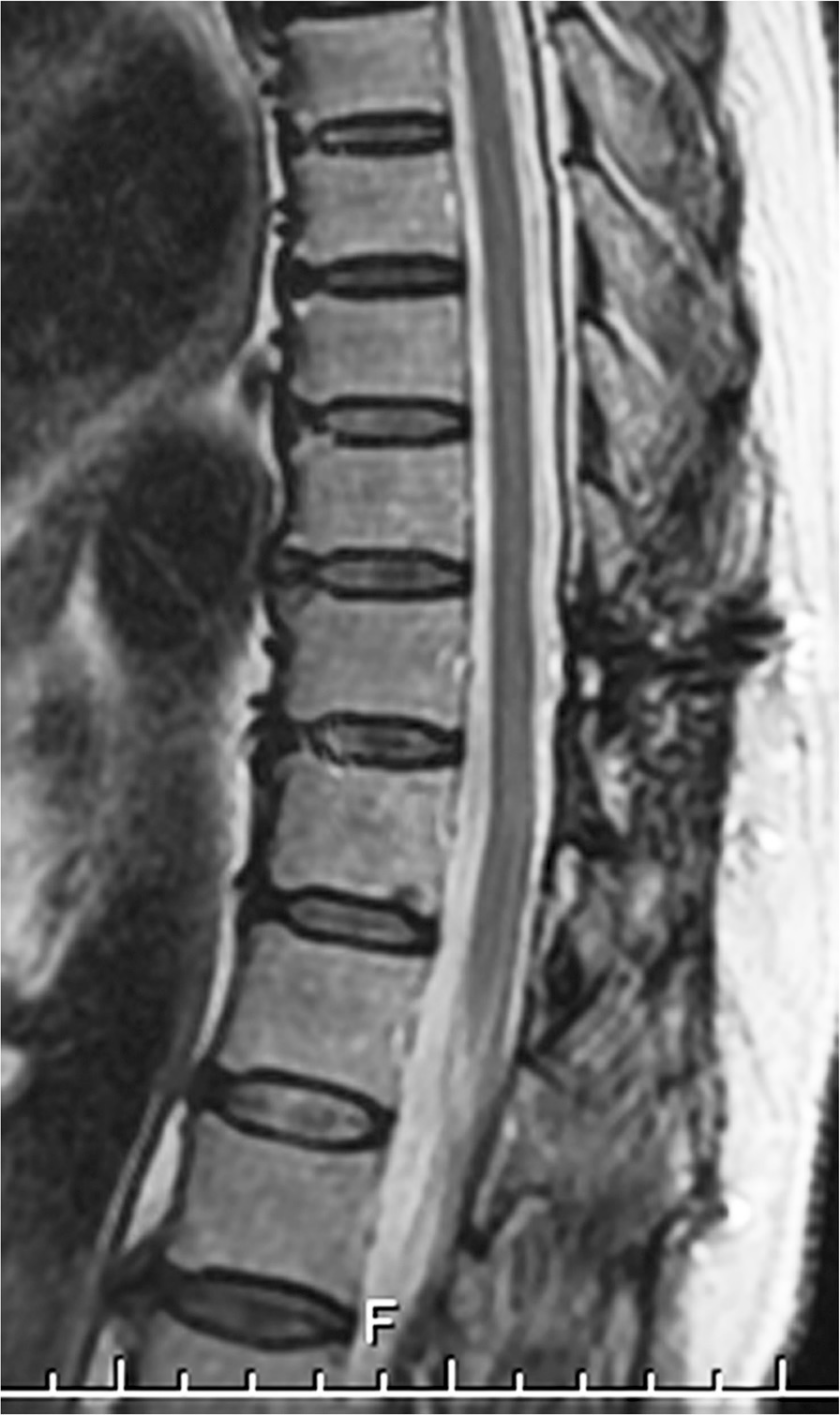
Postoperative sagittal T2-weighted imaging obtained 5 years after surgery. No recurrence of the tumor is evident
